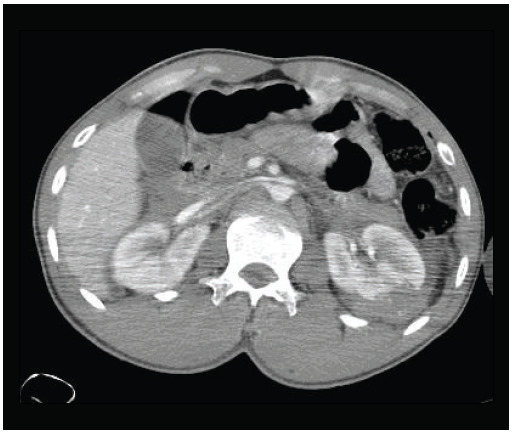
Computed tomography (CT) in Case 2: grade III left renal injury.
